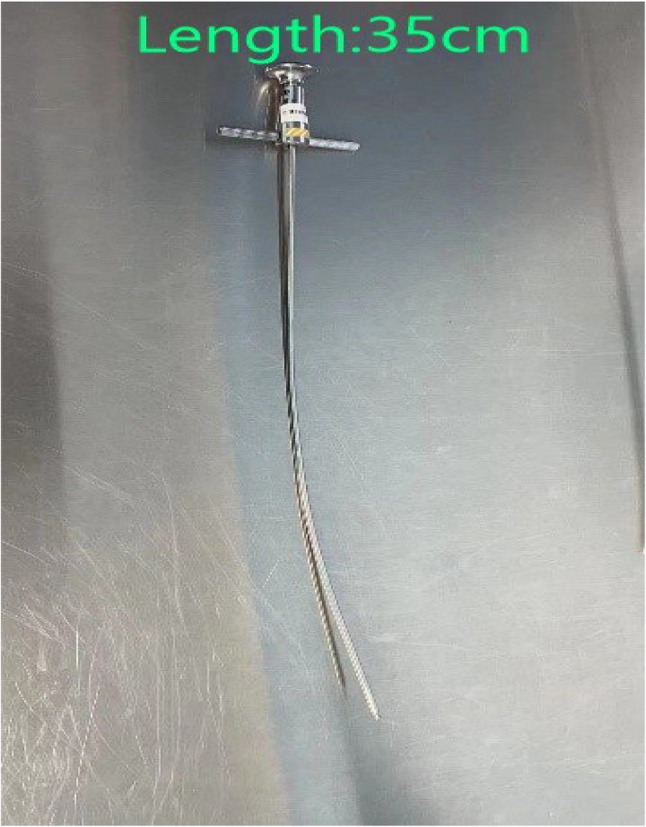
The postoperative CT reveals blood accumulation between the pericardium and the ribs and indicates the possibility of intraoperative injury. The white arrow indicates the position of the catheter. The black arrow indicates the location of the accumulated blood
